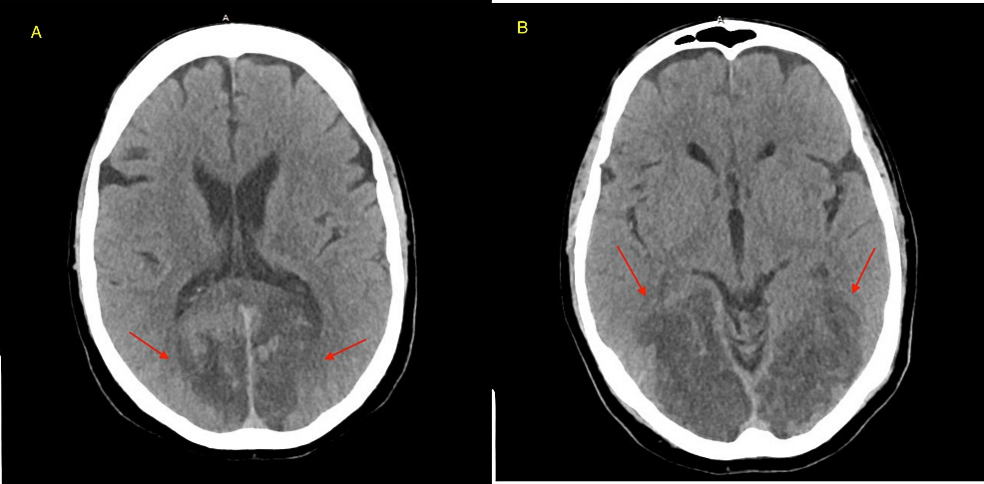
Subsequent CT of the head without IV contrast on day 1 showed ventricles and sulci are normal. No extra-axial fluid collection or subarachnoid hemorrhage is seen. There is no intraparenchymal hematoma or mass. There is loss of gray-white differentiation of the right and left occipital lobes (red arrows in A and B). There is loss of gray-white differentiation of the cerebellum on the right and left, where multiple large acute appearing infarcts are apparent. Dural sinuses are normal in attenuation. Osseous structures are unremarkable. No air-fluid level is noted.
